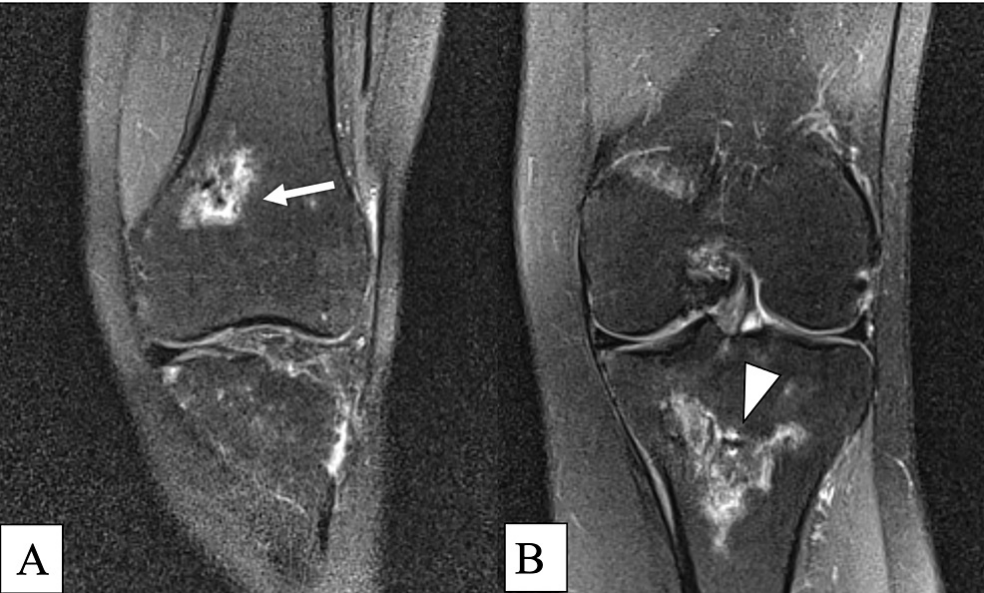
1.5 Tesla MRI dated August 2020 Proton density fat saturation without contrast; coronal view demonstrates the unchanged size of bone infarcts but shows an interval decrease in surrounding bone marrow edema in both the distal femoral metaphysis (arrow) (A) and the proximal tibial metaphysis (arrowhead) (B) MRI: magnetic resonance imaging
